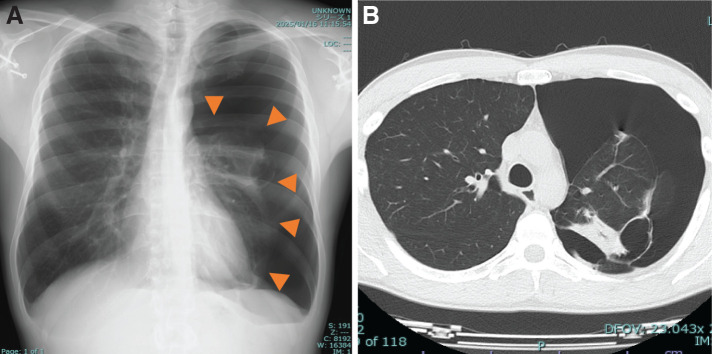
Radiographic findings. (A) Chest radiography demonstrated a large left-sided pneumothorax (arrowheads). (B) Preoperative chest CT revealed subpleural bullae at the left lung apex as well as in the left lower lobe.
